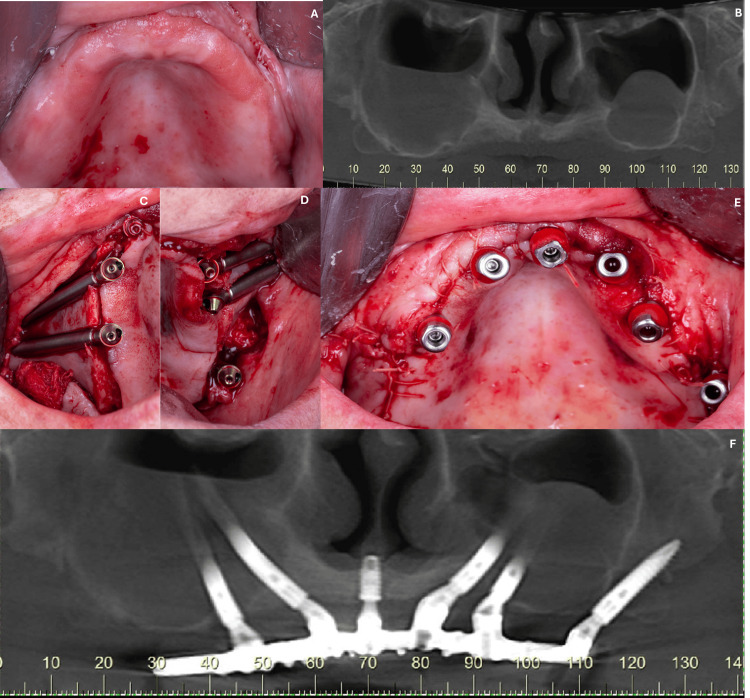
(A) Initial clinical condition; (B) initial radiograph exam showing the atrophic bone condition of the maxilla; (C, D) surgery was performed to place the dental implants; (E) clinical condition after implant placement; (F) radiographic condition of the prosthesis supported by implants after 10 months of follow-up
